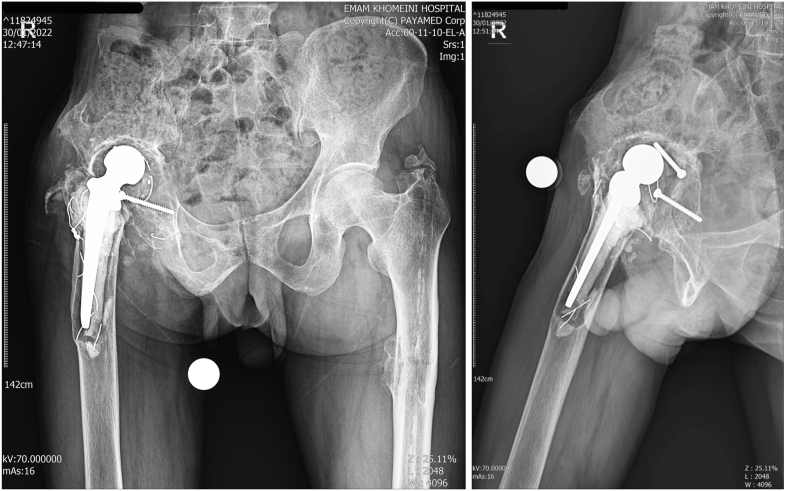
Preoperative radiograph of case 2.
